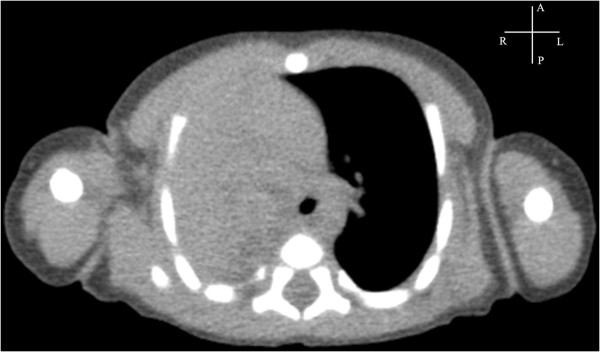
Computed tomography of the same neonate in Figure7showing right lung agenesis.
